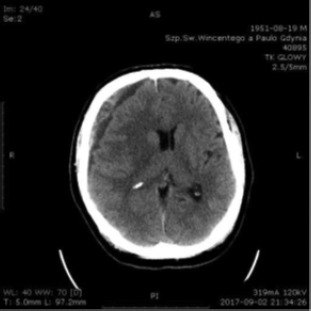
Head CT scan September 2^nd^, 2017
